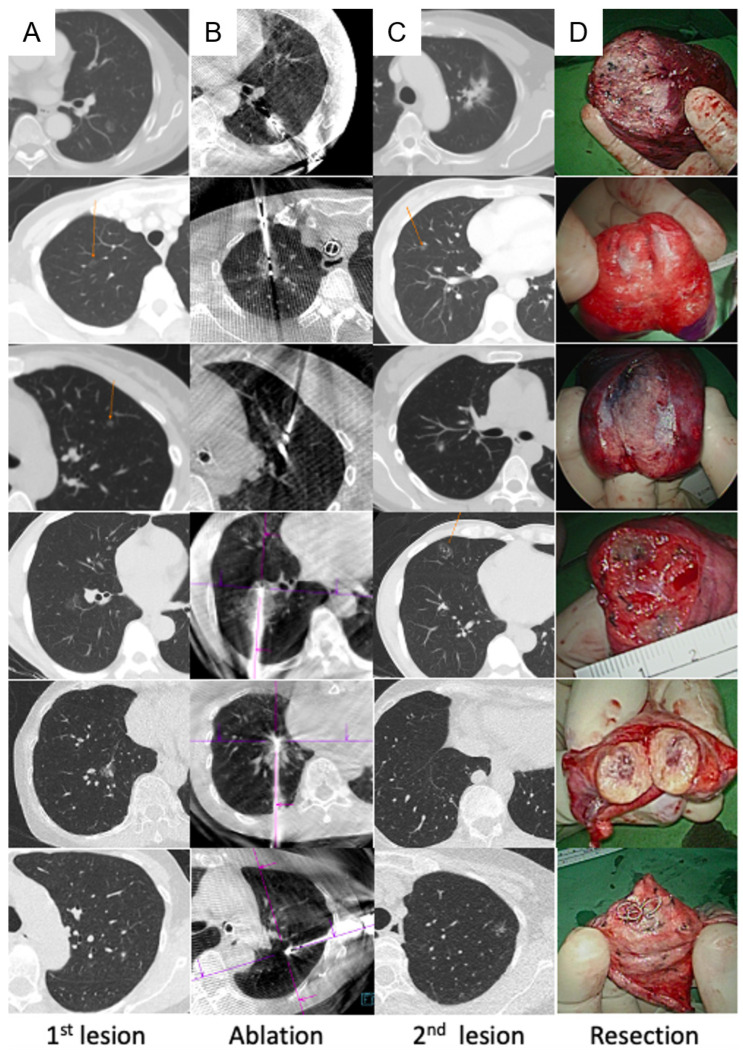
Demonstration of six cases from pre-ablation to resection. (A) Pre-ablation CT scan to indicate the location of the lesion to undergo ablation; (B) post-ablation CT showing the ablation zone and ablation needle in the zone; (C) CT to indicate the location of the lesion to undergo resection; (D) resected part of the lung showing that the lesion was successfully removed. CT, computed tomography.
